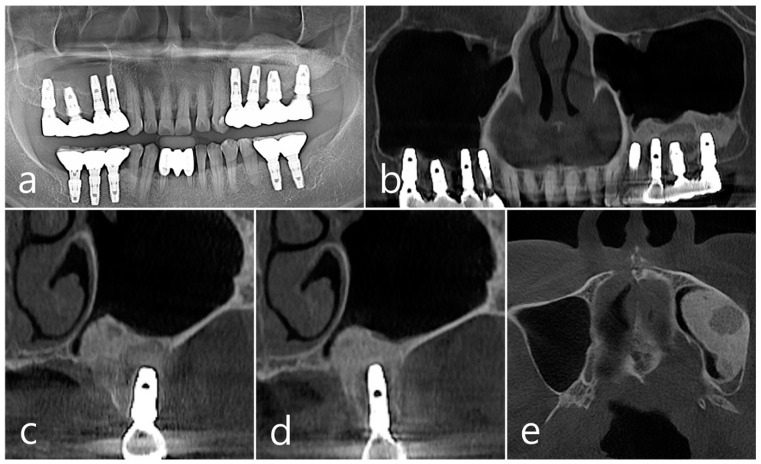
(a,b) In the panoramic radiography and CBCT images taken 1 year after prosthesis delivery, unusual radiolucent images were observed around implants #25 and #26; (c,d) Radiolucent images around #26 and #25 implants were observed. The buccal cortical plate was lost. However, the appearance of the sinus graft was well maintained, and sinus mucosal thickness was normal; (e) A circular radiolucent finding was observed in the axial image of CBCT.
